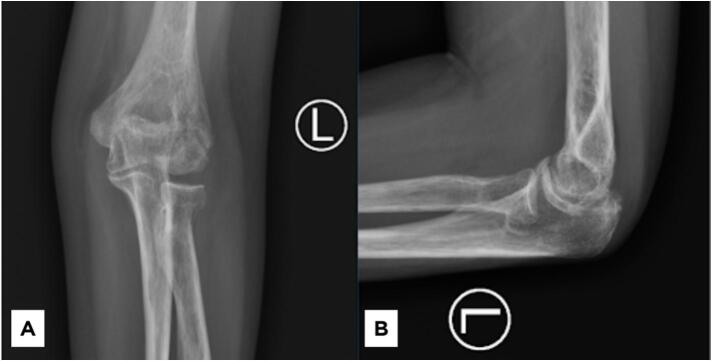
A one-year post-operative X-ray in the AP and lateral positions shows no osteolytic reaction around the insertion of the pin.
